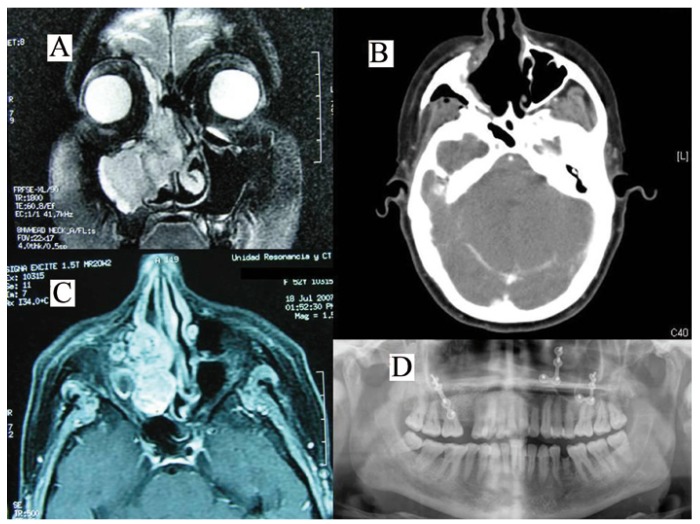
A,C) Craniofacial coronal and axial views showing metachronic metastasis of a previously operated hypernephroma. B, D) Axial CT view and orthopantomography after tumour excision.
